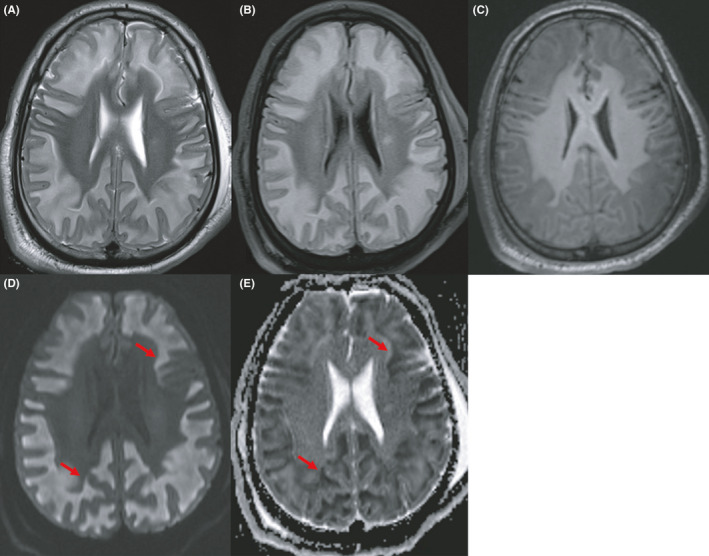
Axial brain magnetic resonance images showing diffuse subcortical white matter demyelination: (A + B) T2‐weighted image (A) and FLAIR sequence (B) showing bilateral diffuse subcortical white matter high signal, sparing subcortical U‐fibers and deep periventricular white matter. (C) Hypointense subcortical white matter on T1‐weighted image. (D + E) This extensive demyelination shows high signal intensity on B1000 diffusion‐weighted image (D) with low apparent diffusion coefficient (ADC) values (arrows)
